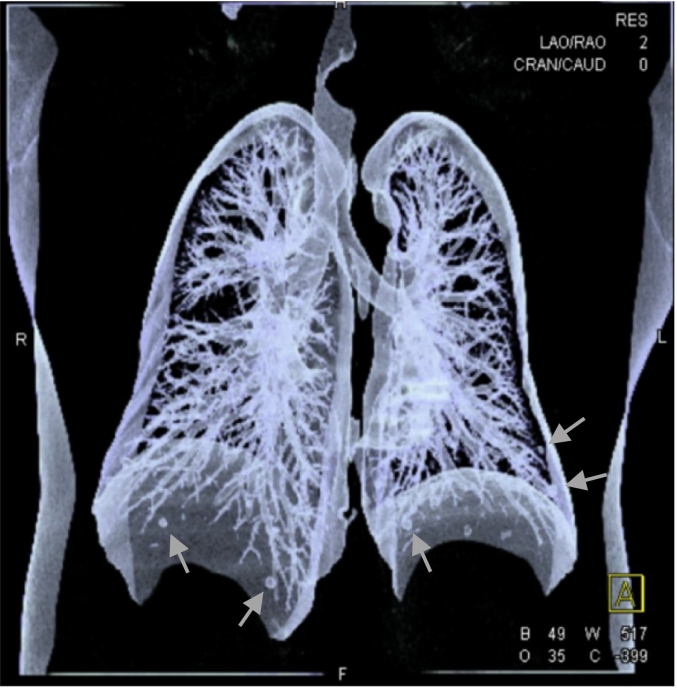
CT scan of the chest: demonstrating several nodules (green arrows). (For interpretation of the references to colour in this figure legend, the reader is referred to the web version of this article.)
